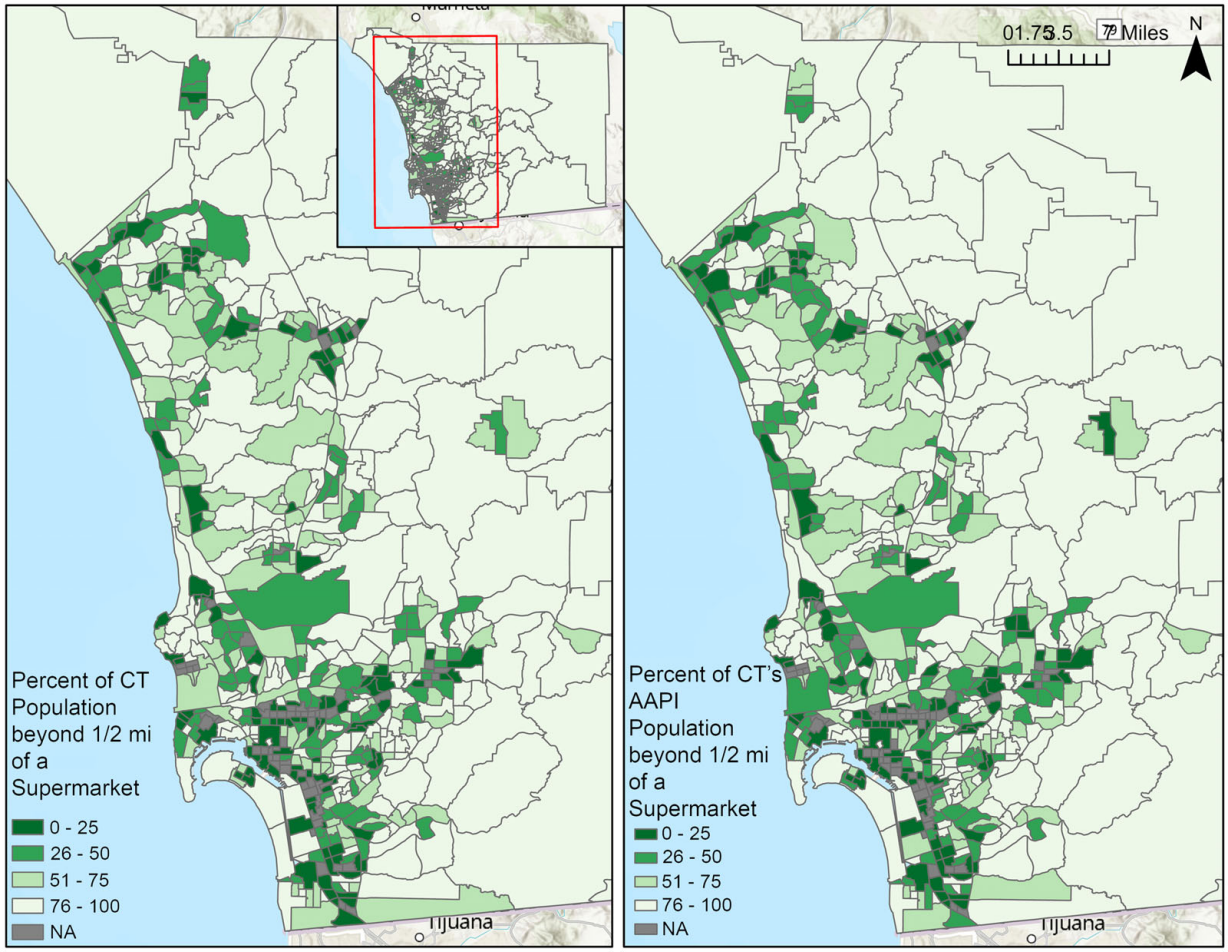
Food Access Research Atlas (FARA) variables: the percent of census tract (CT) population beyond ½ mile from a supermarket (FARA_CT, left), and the percent of CT’s Asian American and Native Hawaiian or Pacific Islander (AAPI) population beyond ½ mile from a supermarket (FARA_AAPI, right).
